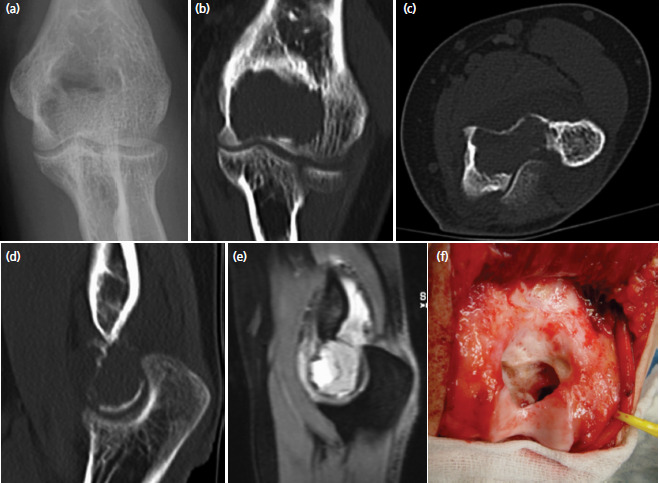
Pre- and intra-operative images revealing the big lytic lesion in the epiphysis of the left elbow: (a) anteroposterior radiograph, (b) coronal, (c) axial and (d) sagittal CT-images, (e) sagittal MRI, and (f) posterior view after intralesional curettage and high-speed burring. The subchondral bone of the trochlea and the medial epicondyle was severely thinned out and posteriorly disrupted. The ulnar nerve was marked with a vessel loop.
